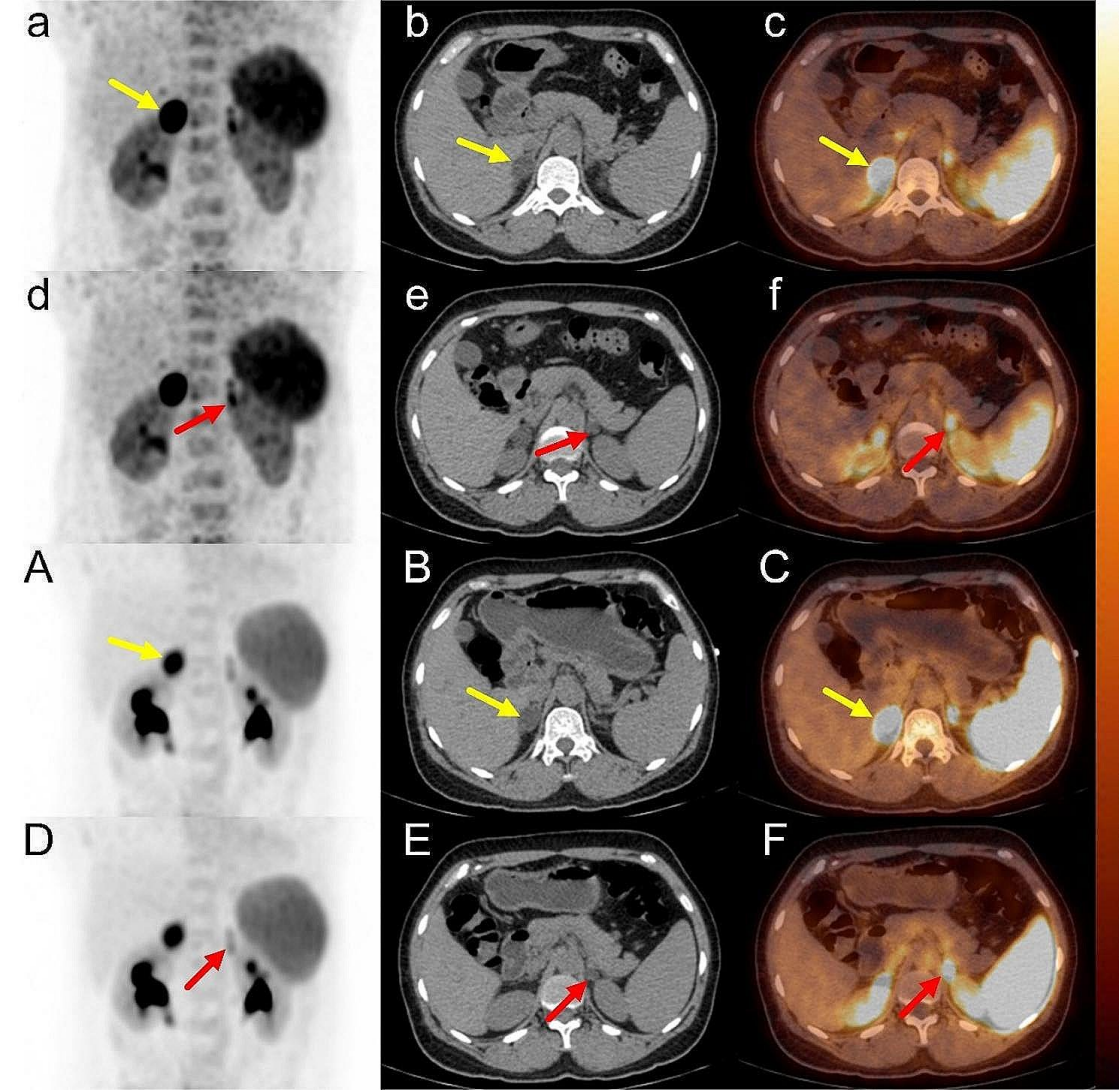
Patient 8 was complicated with SCS. The level of cortisol after 1 mg dexamethasone inhibition test was 115.06 nmol/L, and 24 h-free urinary cortisol was 523.28 nmol/L, with no typical sign of Cushing syndrome. Both 10-min and 40-min ^68^Ga-pentixafor PET indicated UPA (right) (10 min: a-f; 40 min: A-F), while AVS showed BPA (LI = 1.96). a-c: right adrenal gland, SUVmax = 25.93 (yellow arrow); d-f: left adrenal gland, SUVmax = 8.18 (red arrow); CON = 3.17. A-C: right adrenal gland, SUVmax = 30.39 (yellow arrow); D–F: left adrenal gland, SUVmax = 7.10 (red arrow); CON = 4.28.
